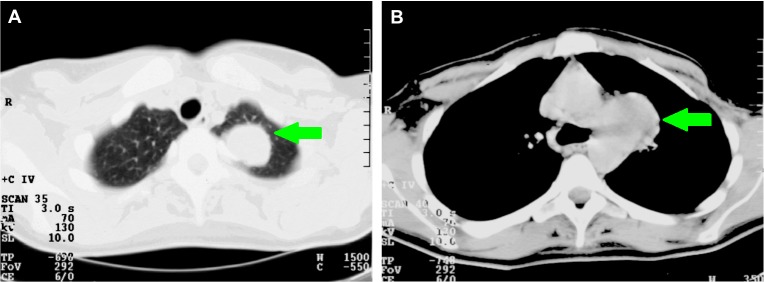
Axial computed tomography (CT) scan in January 1996 pre-treatment. A. The CT scan shows a mass in the left upper lobe of the lung. B. The CT scan shows a large mass in the aortic pulmonary window, reaching from the lateral side of the aortic arch to the left and right pulmonary arteries.
